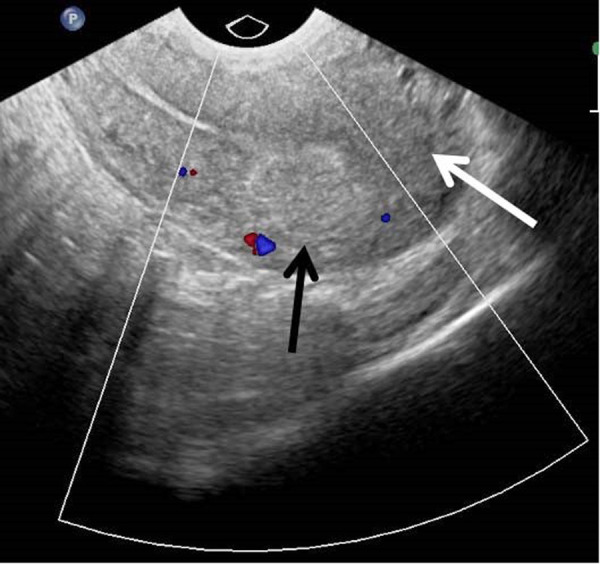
Transvaginal colour Doppler ultrasound image of the uterus in sagittal plane one month after embolisation demonstrates resolution of previous vascular lesion (white arrow), while hyperechoic mass within myometrium (black arrow) indicates embolic agent left post selective embolisation
